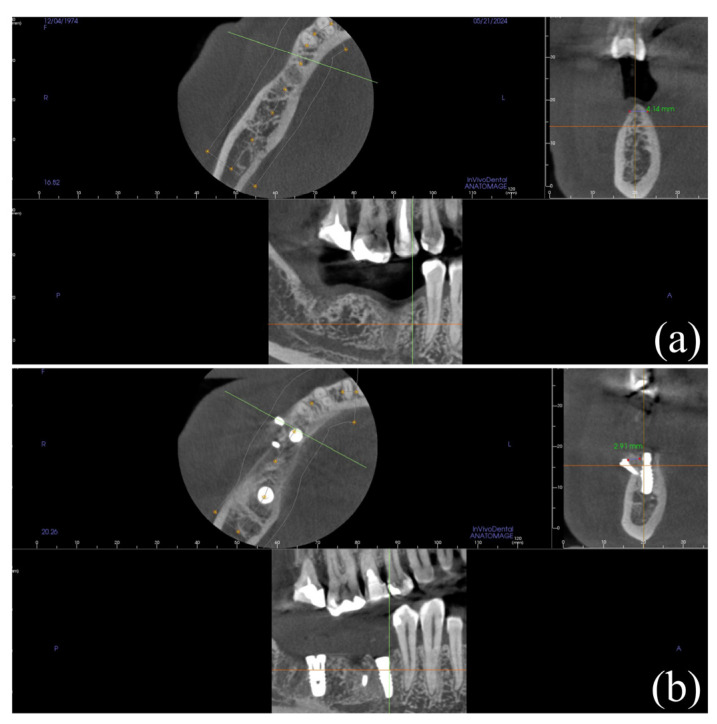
Radiographic evaluation of the clinical case shown in Figure 2. (a) Preoperative radiographic view. (b) Postoperative radiographic view after implant placement and augmentation. Colored lines are used only as visual reference markers and do not represent additional quantitative measurements.
